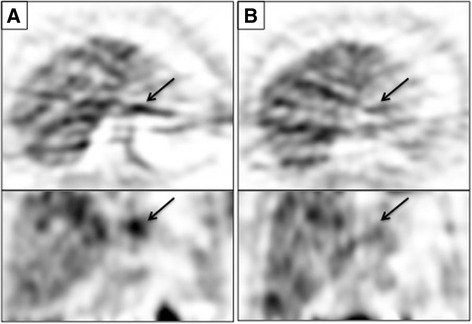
Tumor ^18^F-FAU Uptake in Patient 15. Axial (top) and coronal (bottom) ^18^F-FAU Images of a tumor of the gastroesophageal junction (arrow) at baseline (a) and after 1 day of capecitabine therapy (b). Tumor SUV_mean_ decreased from 3.47 to 2.07
